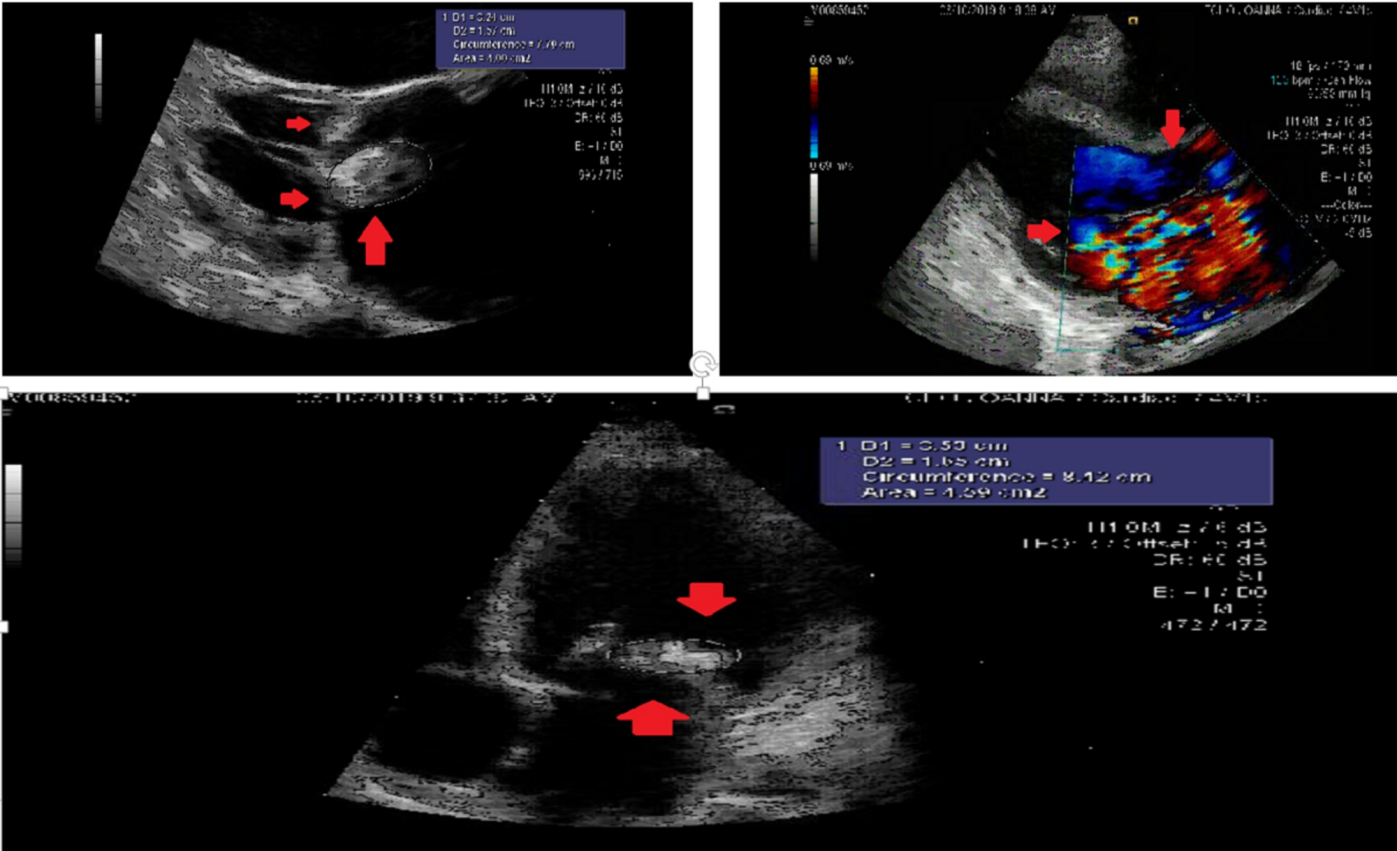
Transthoracic echocardiogram parasternal long axis view on the top showing mobile posterior leaflet vegetation (top left) and severe mitral regurgitation jet (top right), and at the bottom showing apical four chamber view with large vegetation on the posterior leaflet of mitral valve
